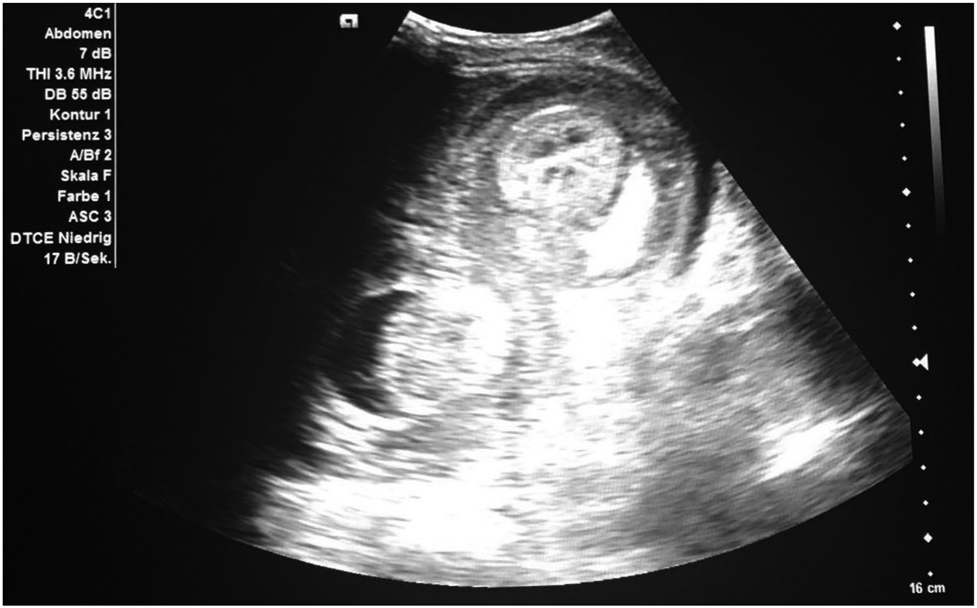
Bedside abdominal ultrasound scan revealed “target sign”.
